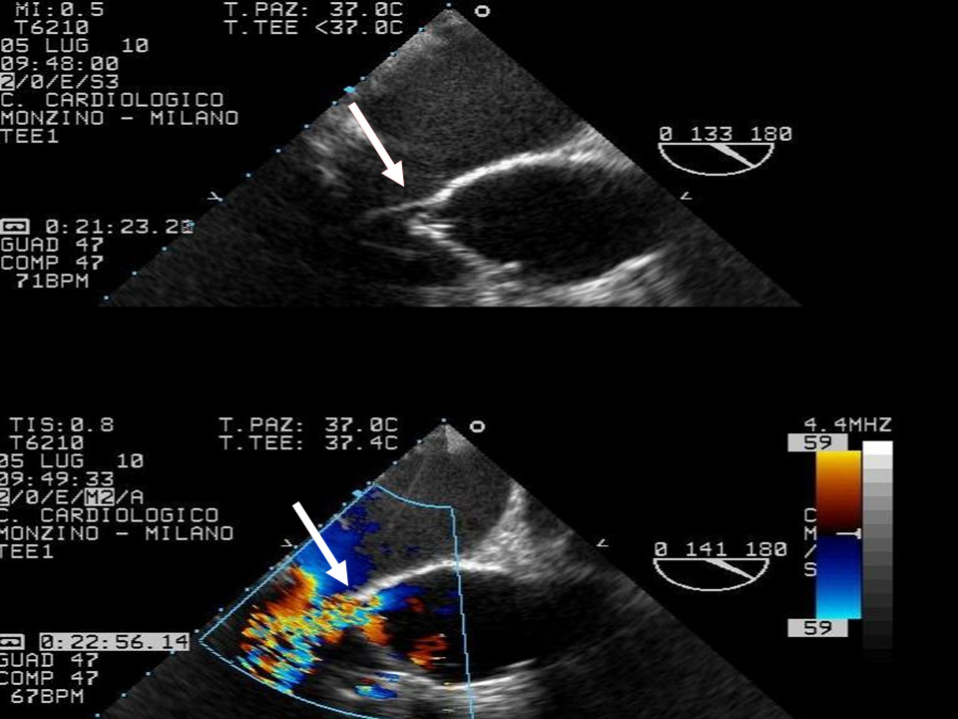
The transesophageal echocardiogram shows severe aortic prosthetic regurgitation (white arrow), due to significant paravalvular leakage in the non-coronary aortic sinus.
